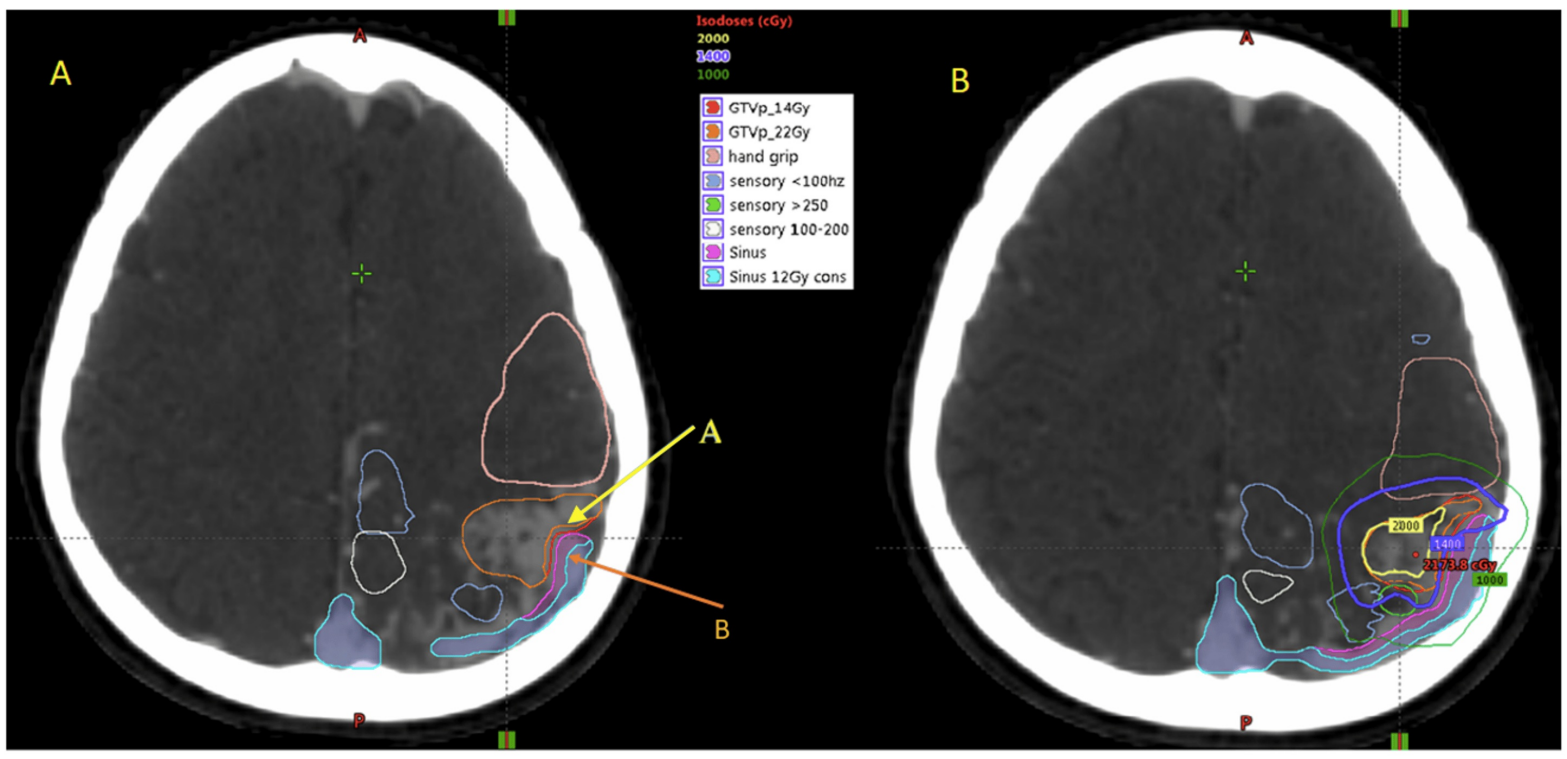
Contours on axial CT images after co-registration with MRI and CT angiogram. (A) Co-registered contours with two different prescription isodoses for the GTV (arrow A) and two different constraints for lateral sinus (arrow B). (B) Isodose lines generated by the treatment planning system showing a 14Gy isodose line (blue) covering the full GTV and 20Gy isodose line (yellow) covering the GTV with safety margin from lateral sinus.
